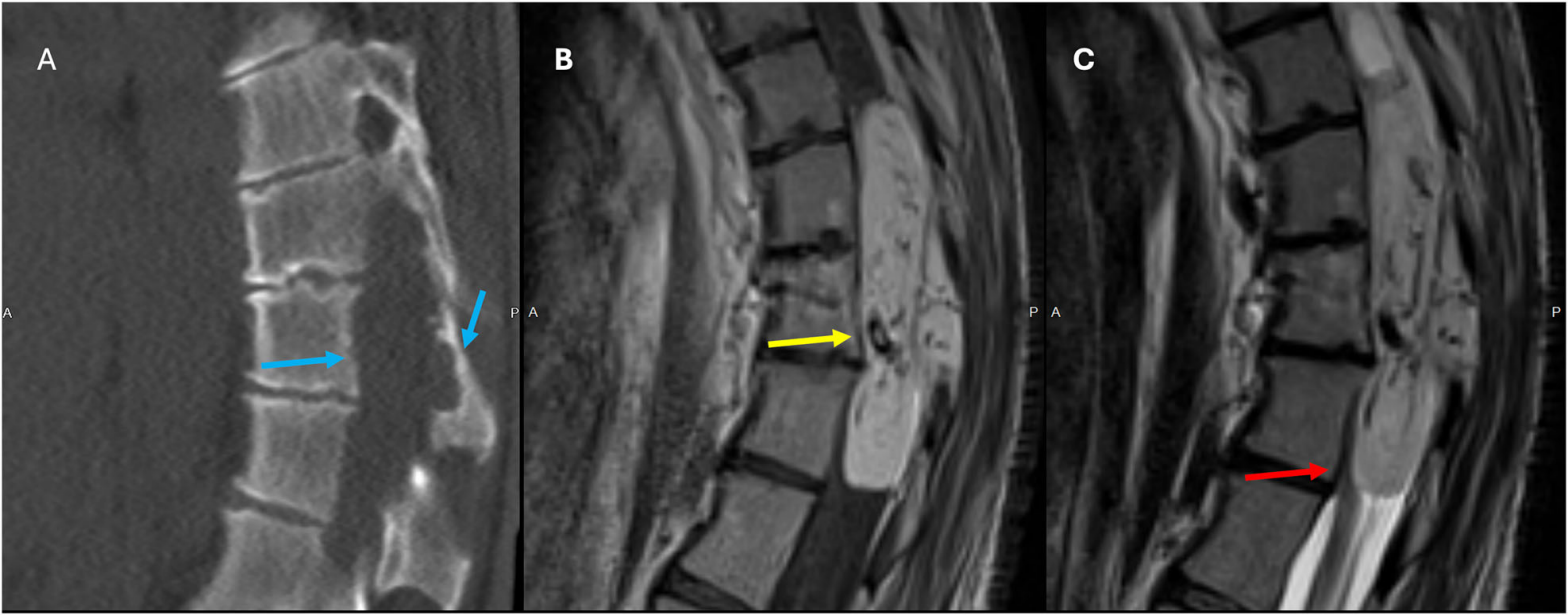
36-year-old patient with a history of cerebral palsy, admitted for urinary retention and sepsis. A Unenhanced CT of the spine showing an isodense expansile mass lesion with posterior vertebral body and posterior elements scalloping (blue arrows). T1 postcontrast (B image), T2W (C image) images showing a large well-defined smooth, expansile mixed signal, heterogeneously enhancing intradural, extramedullary mass arising from the filum terminale and extending from T11 to L2 (yellow arrow). This is truncating the conus and obliterating the central canal with cauda equina compression (red arrow)
